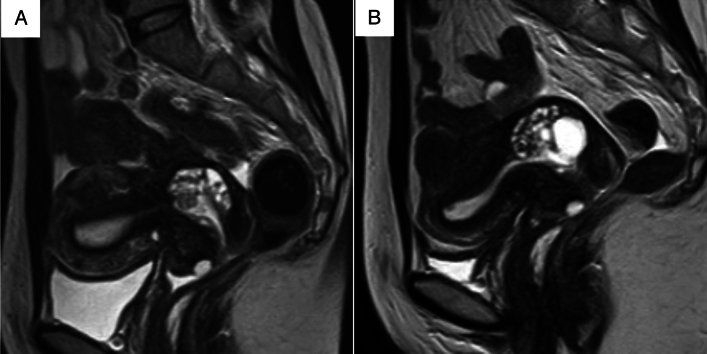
A Sagittal T2-weighted (T2WI) image of a 45-year-old woman with lobular endocervical glandular hyperplasia showing a cystic aggregate lesion in the upper part of the cervix. The lesion is composed of some small cysts and solid components in the central part of the lesion, surrounded by relatively large cysts. This lesion was classified as the “typical cosmos pattern.” B Sagittal T2WI of the same patient at the age of 51 years (premenopausal) shows a cystic aggregate lesion in the upper part of the cervix. The lesion has increased in size, but the typical cosmos pattern remained unchanged
